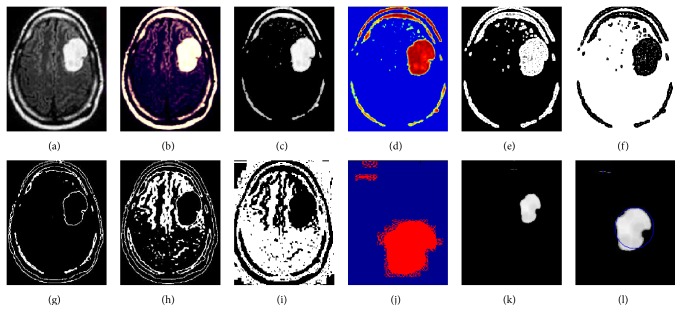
Segmented and area extracted result of brain MR image. (a) Original image. (b) Enhanced image. (c) Skull-stripped image. (d) Wavelet transpose image. (e) Intense segmented image. (f) Inverse intense image. (g) Gray matter. (h) White matter. (i) CSF. (j) Dice overlap image. (k) Eroded image. (l) Area extracted image.
